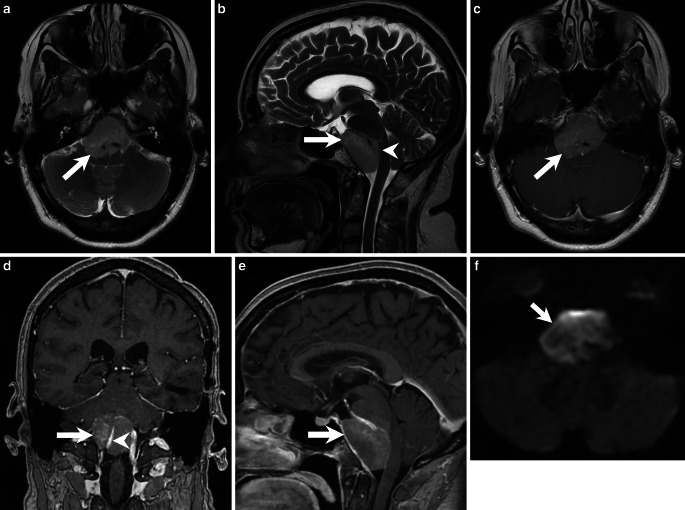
MR imaging 12 months after initial and total resection of the left temporal glioblastoma multiforme. Axial (a) and sagittal (b) T2 weighted images showed a space-occupying lesion (a,b *arrow*) located in the prepontine cistern. The lesion compressed and dislocated the brainstem and the myelon (*arrowhead*). On T1-weighted axial (c), coronal (d), and sagittal (e) MRI after administration of Gd the lesion showed some degree of homogeneous enhancement of contrast. The basilar artery was completely encased by the tumor (d, *arrowhead*). There was dural enhancement along the clivus. Thickening of the meninges as well as any direct relation to the clivus were absent. On diffusion-weighted images (b-value: 1000, f) the lesion (*arrow*) showed restricted diffusion
